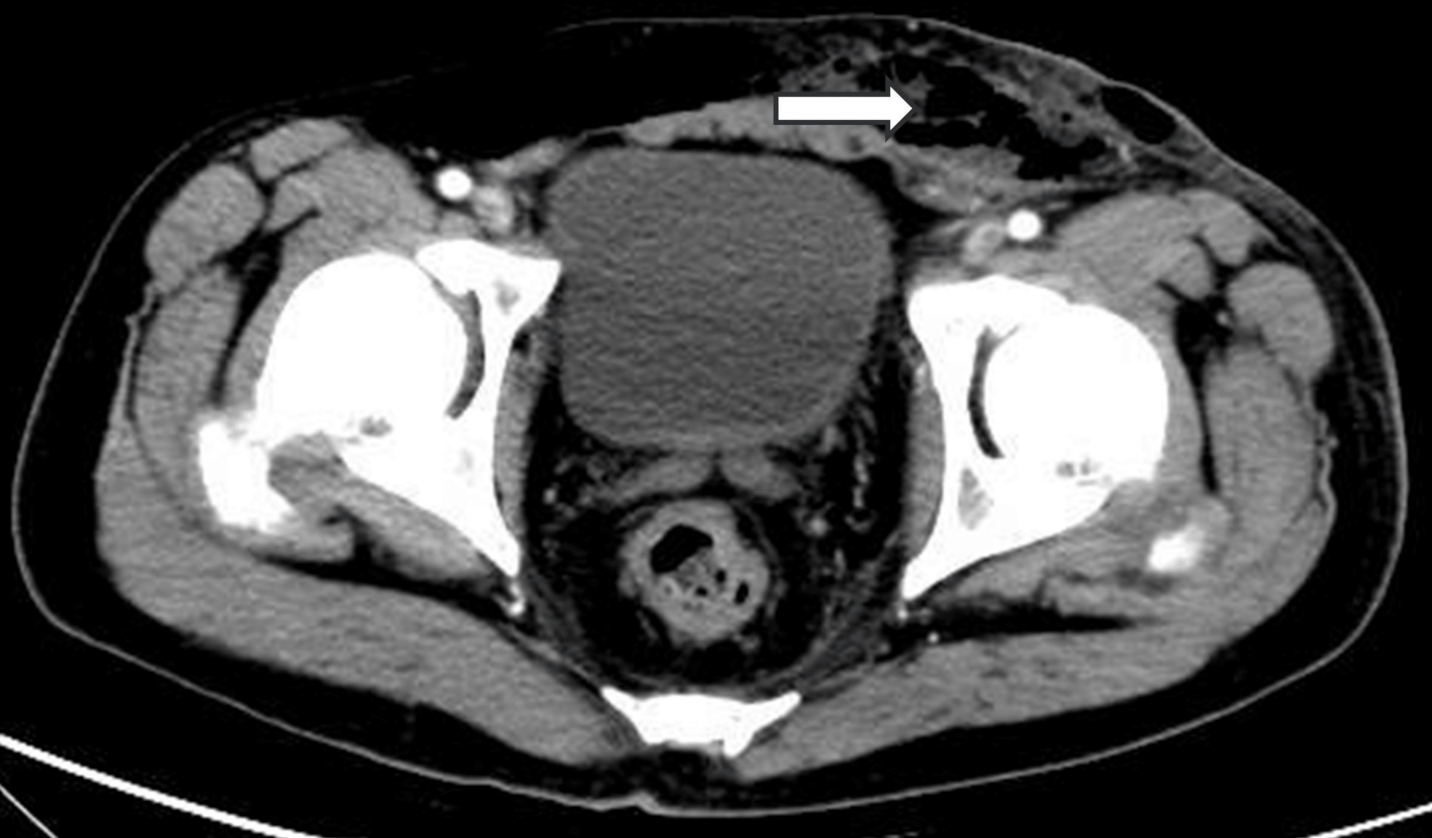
Pelvic CT of the left lower abdominal wall has infections and subcutaneous emphysema.
